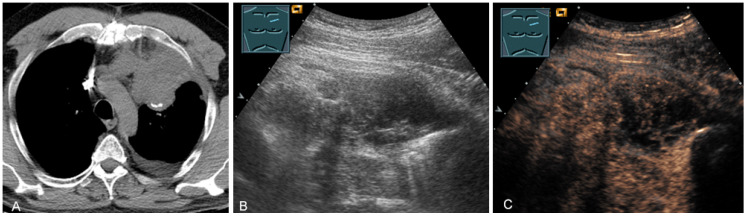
A patient with tumor formation in the anterior mediastinum on CT (A), B-mode US (B), and CEUS (C), with anamnestic osteosarcoma, confirmed by biopsy as mediastinal metastasis.
